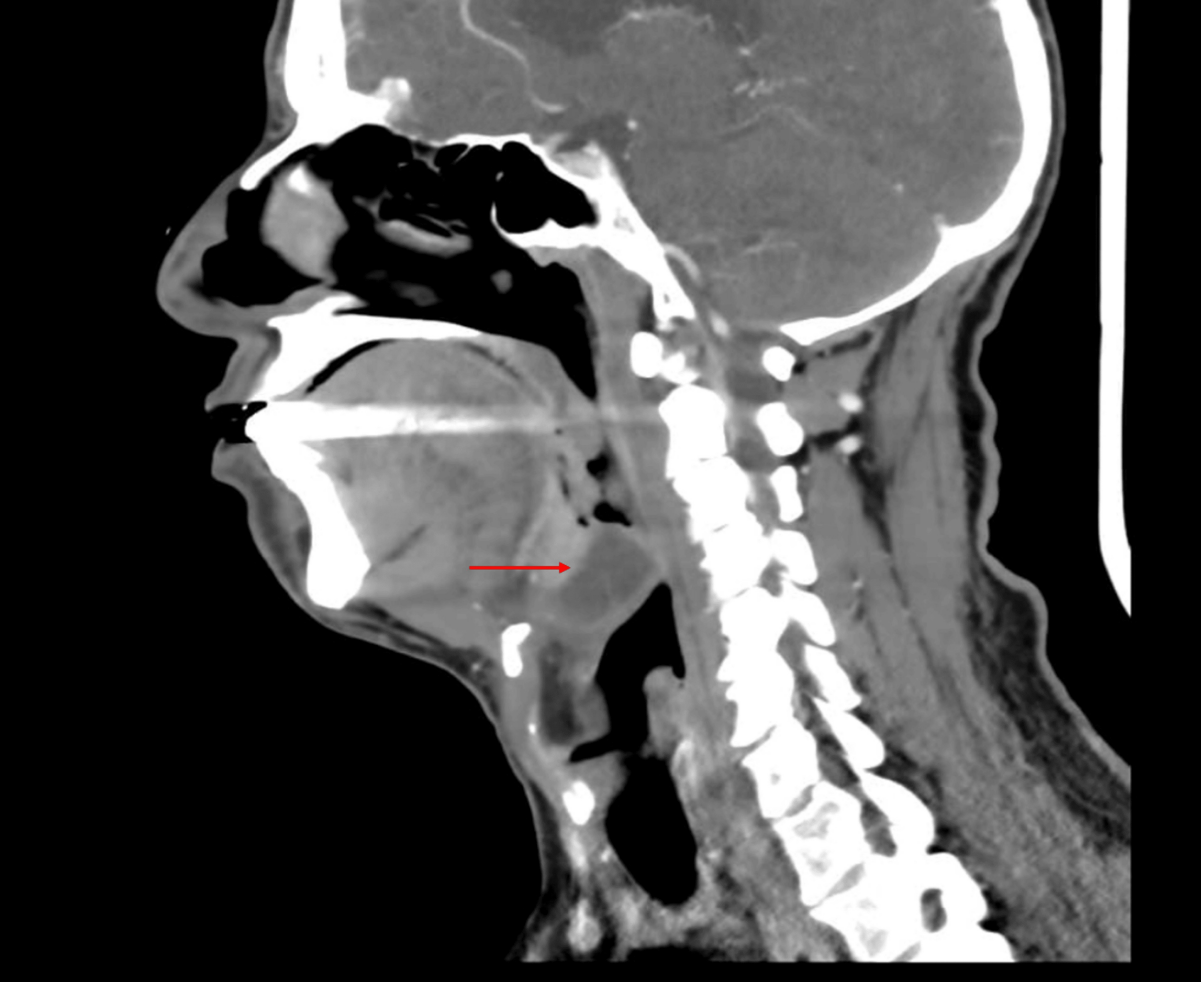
CT with contrast showing epiglottitis and epiglottic abscess
